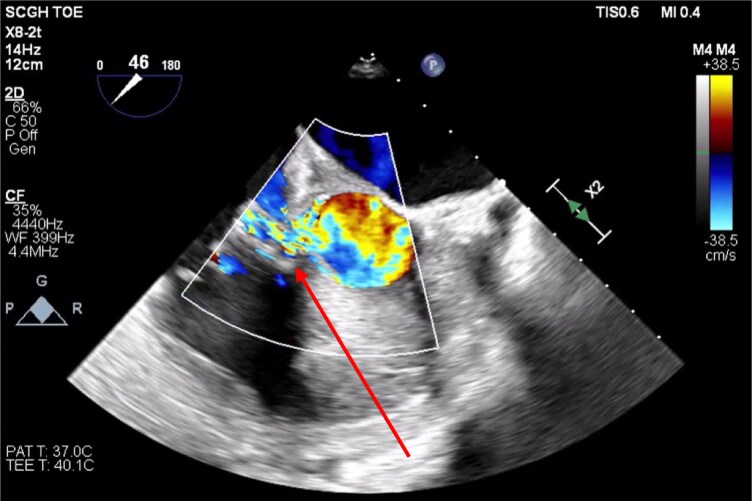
Intraoperative transoesophageal echocardiogram image short axis showing colour Doppler of flow across the Gerbode defect into the right atrium, superior to the tricuspid annulus (red arrow).
